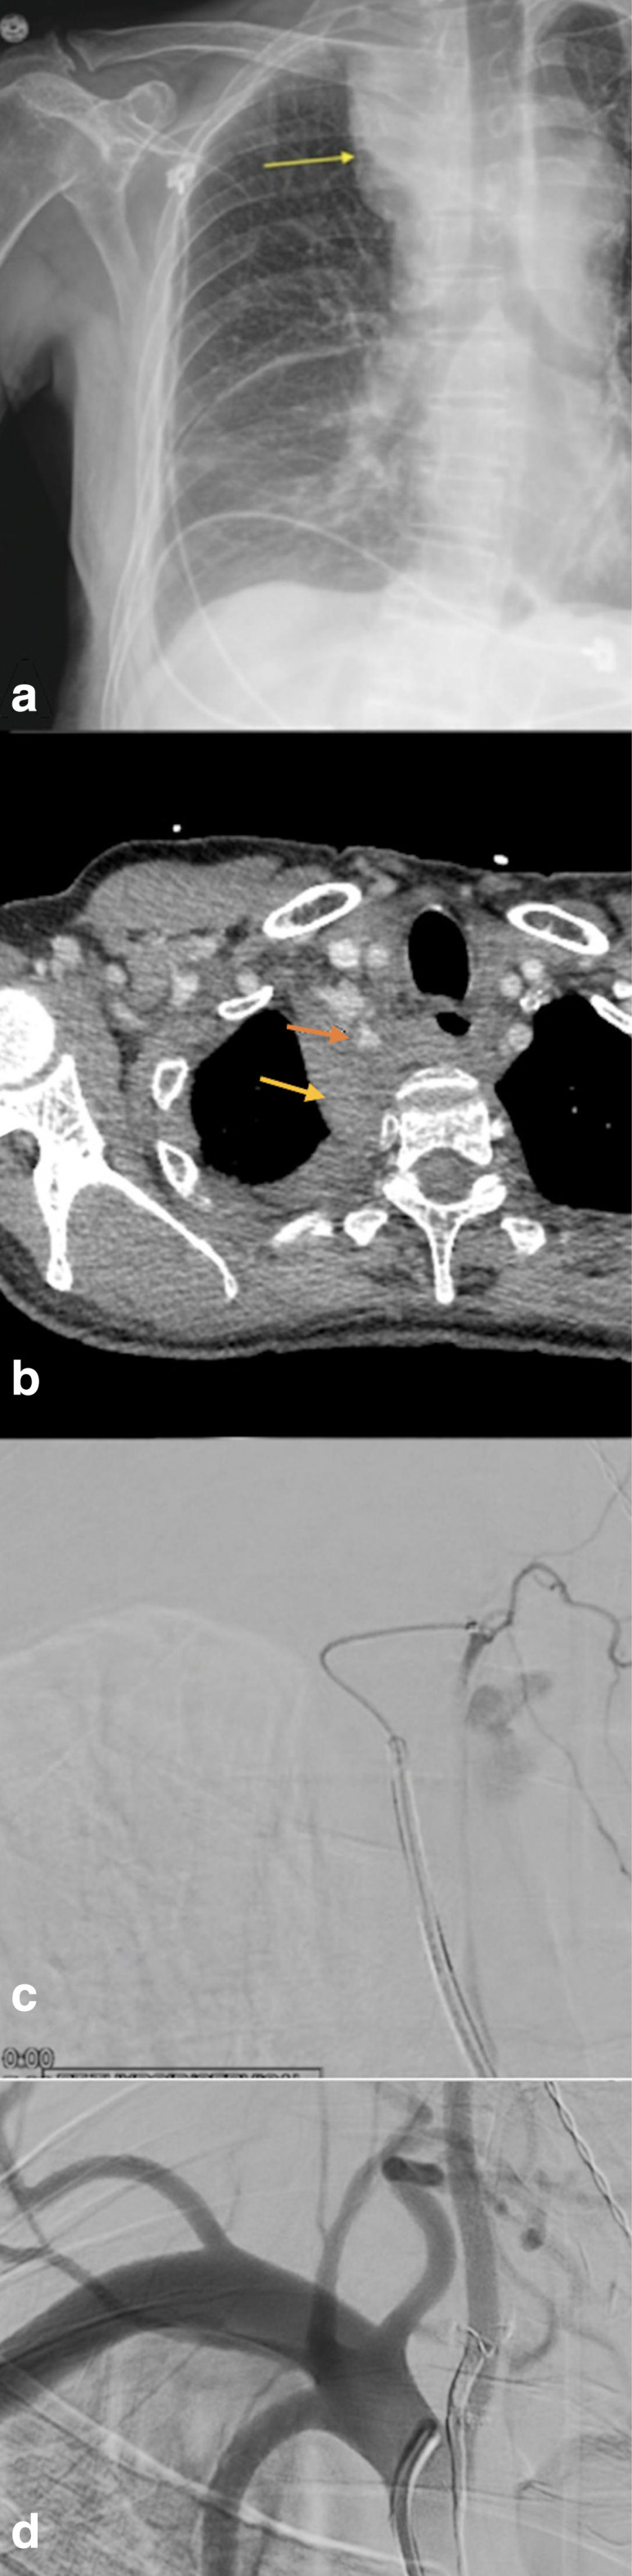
(a) Anteroposterior chest X-ray showing right upper peri mediastinal opacity (Yellow Arrow). (b) Contrast-enhanced chest CT scan demonstrating a right hemomediastinum and right hemothorax (Red Arrows). (c) A superselective injection showing the thyrocervical trunk pseudoaneurysm. (d) Fluoroscopy showing complete obliteration of the pseudoaneurysm after Onyx embolization.
